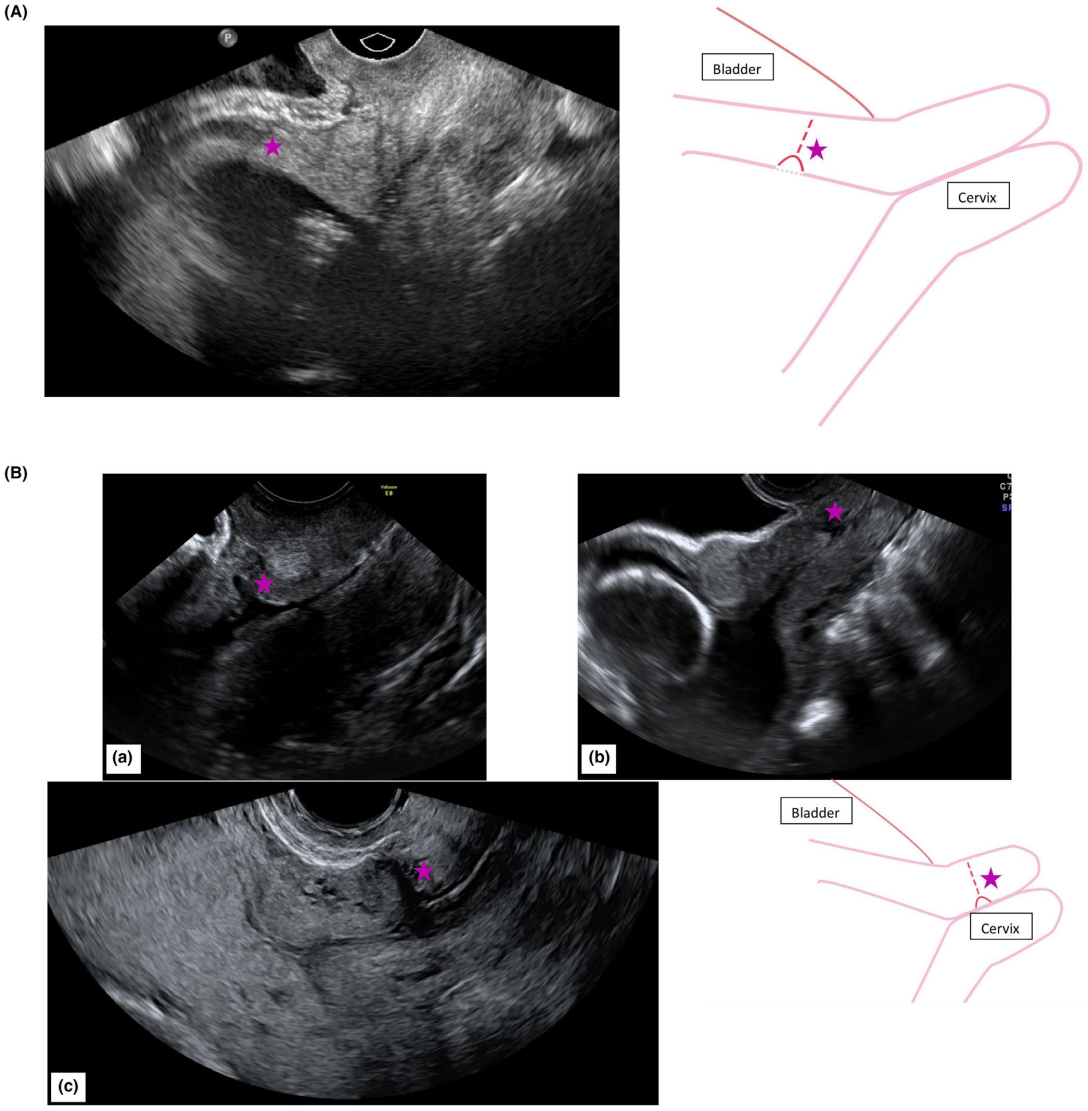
(A) The cesarean scar is intrauterine in a women at 20 weeks of gestational age after having a planned cesarean delivery without any contractions or effaced cervix, as her baby was laying in breech position. (B) Vaginal ultrasound in three different women of (a) 13 weeks of gestational age, (b) 20 weeks of gestational age, (c) 26 weeks of gestational age. The cesarean scar is intracervical due to a secondary cesarean delivery in the medical history. stars, cesarean scars
